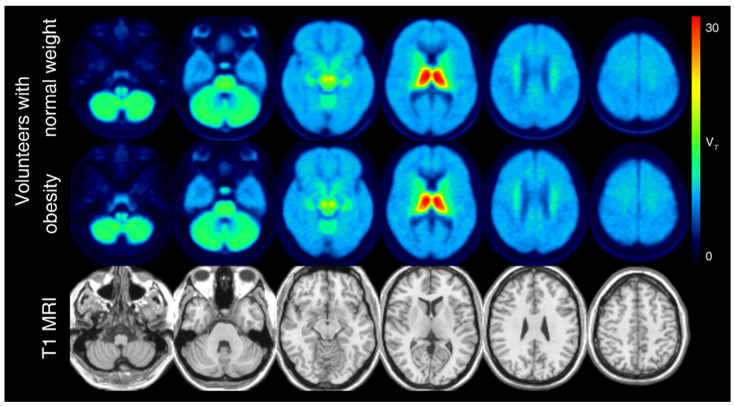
The (−)-[^18^F]flubatine average total distribution volume of all normal-weight volunteers (first row) and volunteers with obesity (second row) using positron emission tomography co-registered to T1 (third row) magnetic resonance imaging.
